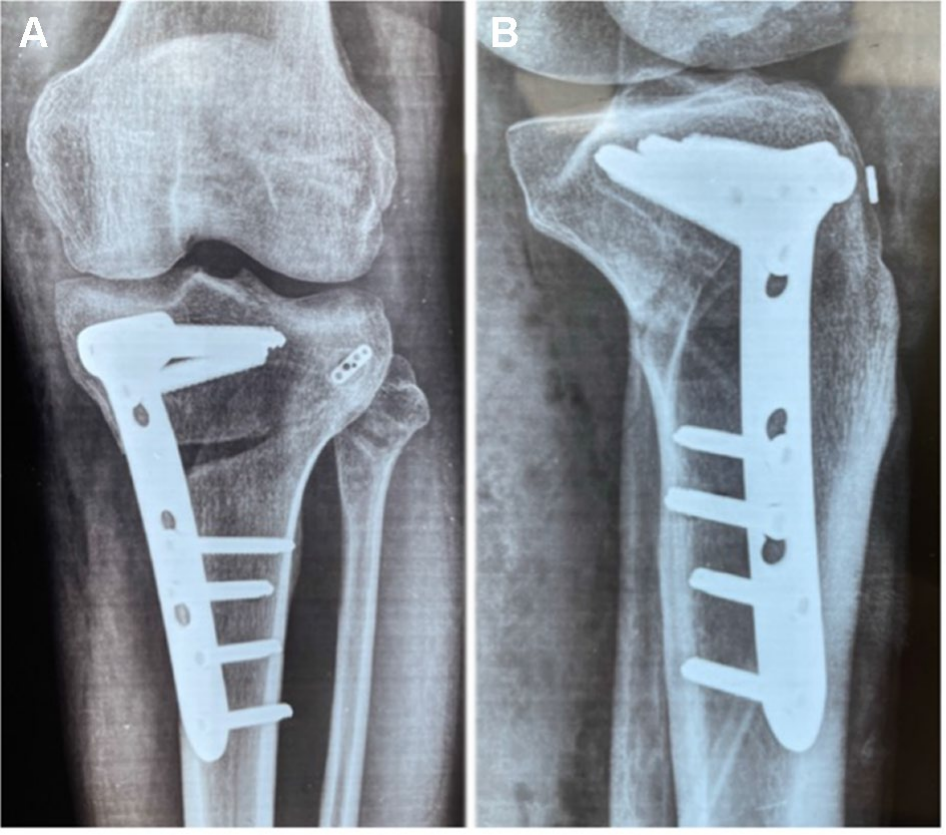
Postoperative X-ray showing concomitant high tibial osteotomy and posterolateral corner reconstruction in a double varus knee with varus thrust gait. A Anteroposterior view. B Lateral view showing the adjustable button sitting flush with the anterolateral aspect of the proximal tibia
